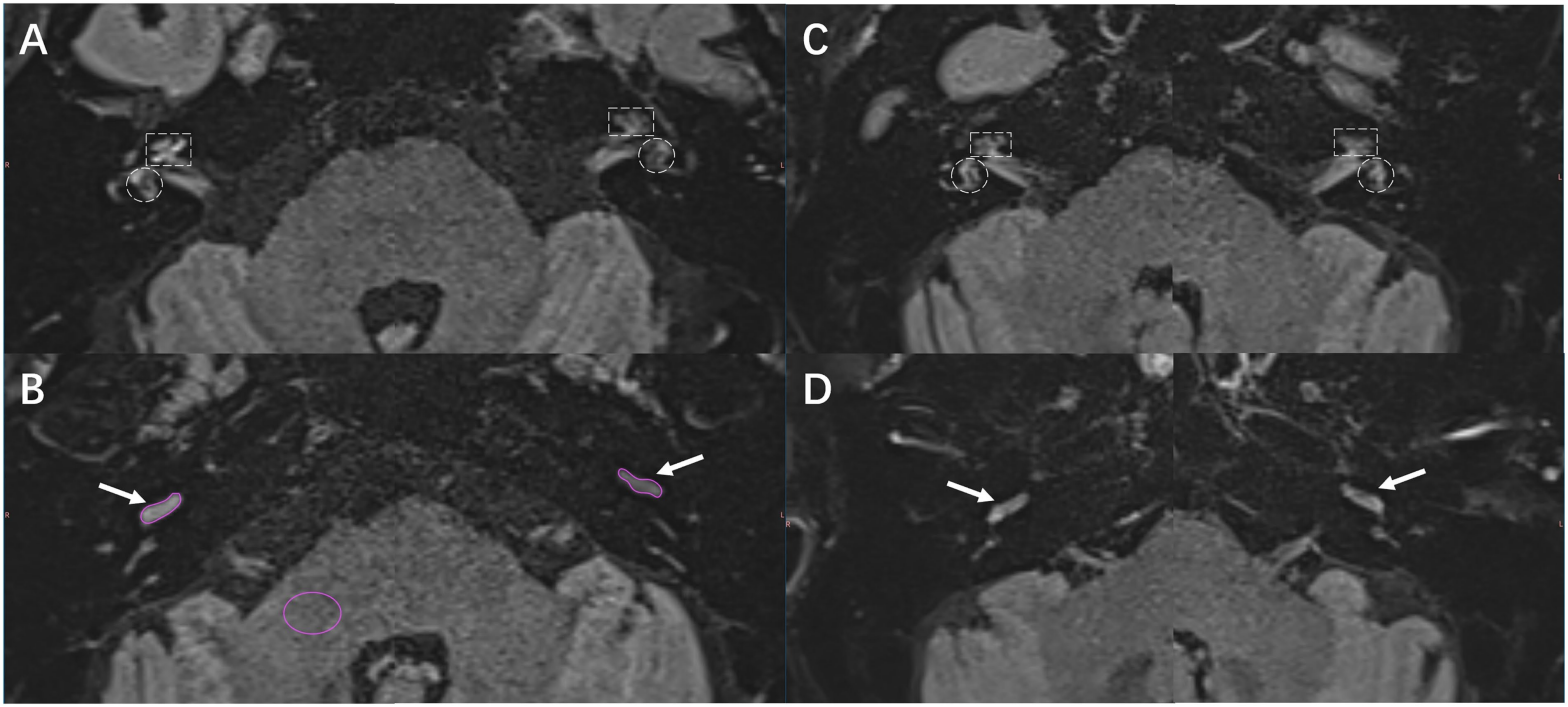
presents representative gadolinium-enhanced inner ear MRI images from patients diagnosed with Ménière’s disease (MD) and vestibular migraine (VM). (A,B) The images depict a 54-year-old male patient with MD. (A) Notable perilymph enhancement is observed in the cochlear basal turn of the right (ipsilateral) ear, characterized by grade 2 cEH and grade 2 vEH. In contrast, the left (contralateral) ear displays grade 1 cEH without vestibular hydrops. (B) The SIR measurement involved manually delineating ROIs on the cochlear basal turn and a 0.5 cm^2^ pontine area within the same slice. The SIR was computed as the ratio of mean cochlear signal intensity to mean pontine signal intensity. (C,D) The images of a 72-year-old female patient with VM are shown. (C) Bilateral grade 1 cEH is observed, with grade 1 vEH present on the left and no significant vEH on the right. (D) Symmetrical perilymph enhancement is noted in both cochlear basal turns. In all panels, the white dashed rectangles represent the cochlea, white dashed circles denote the vestibule, white arrows indicate the cochlear basal turn, and purple contours outline the ROIs for SIR measurement.
